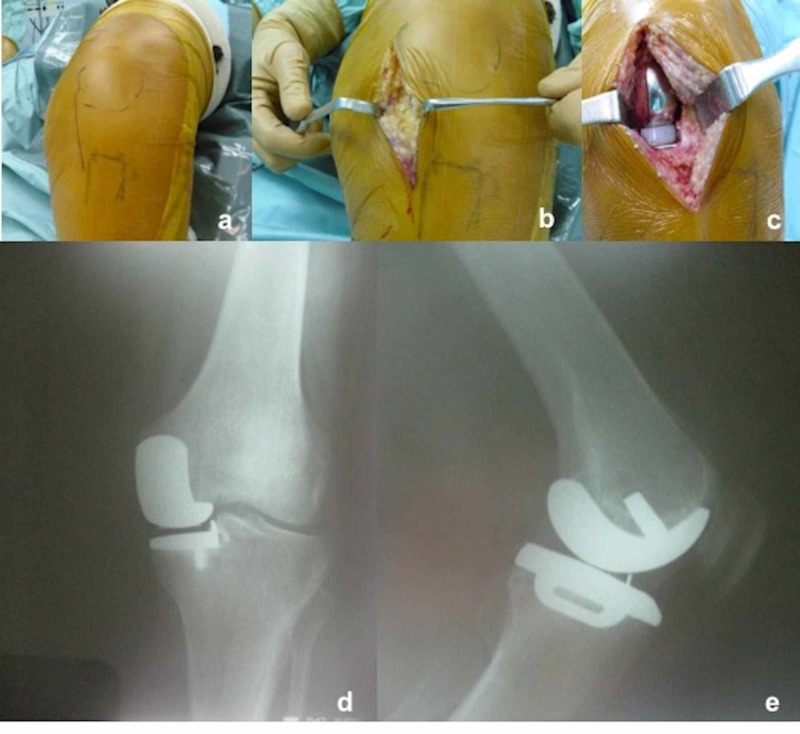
a) Incision marked after draping, b) medial parapatellar incision and arthrotomy, c) placement of the implants, and d,e) postoperative anteroposterior/lateral radiographs of the knee
